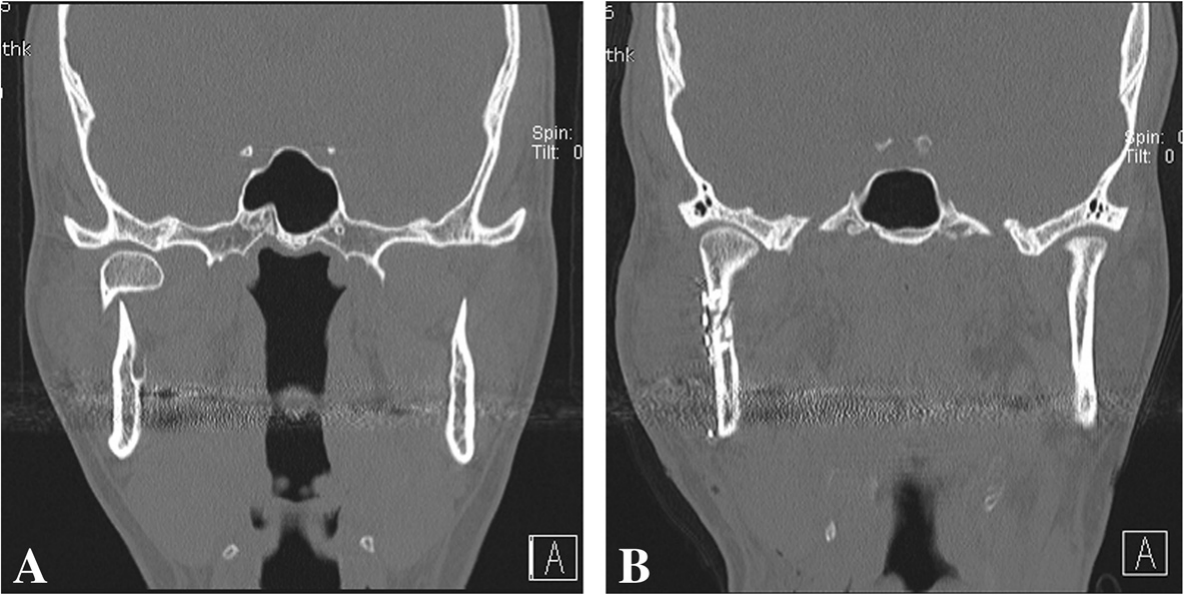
A 33-year-old male patient with a right condylar neck fracture. A. Preoperative CT findings. B. Reduction and fixation of miniplates was performed with the use of a trochar combined with the Risdon approach.
